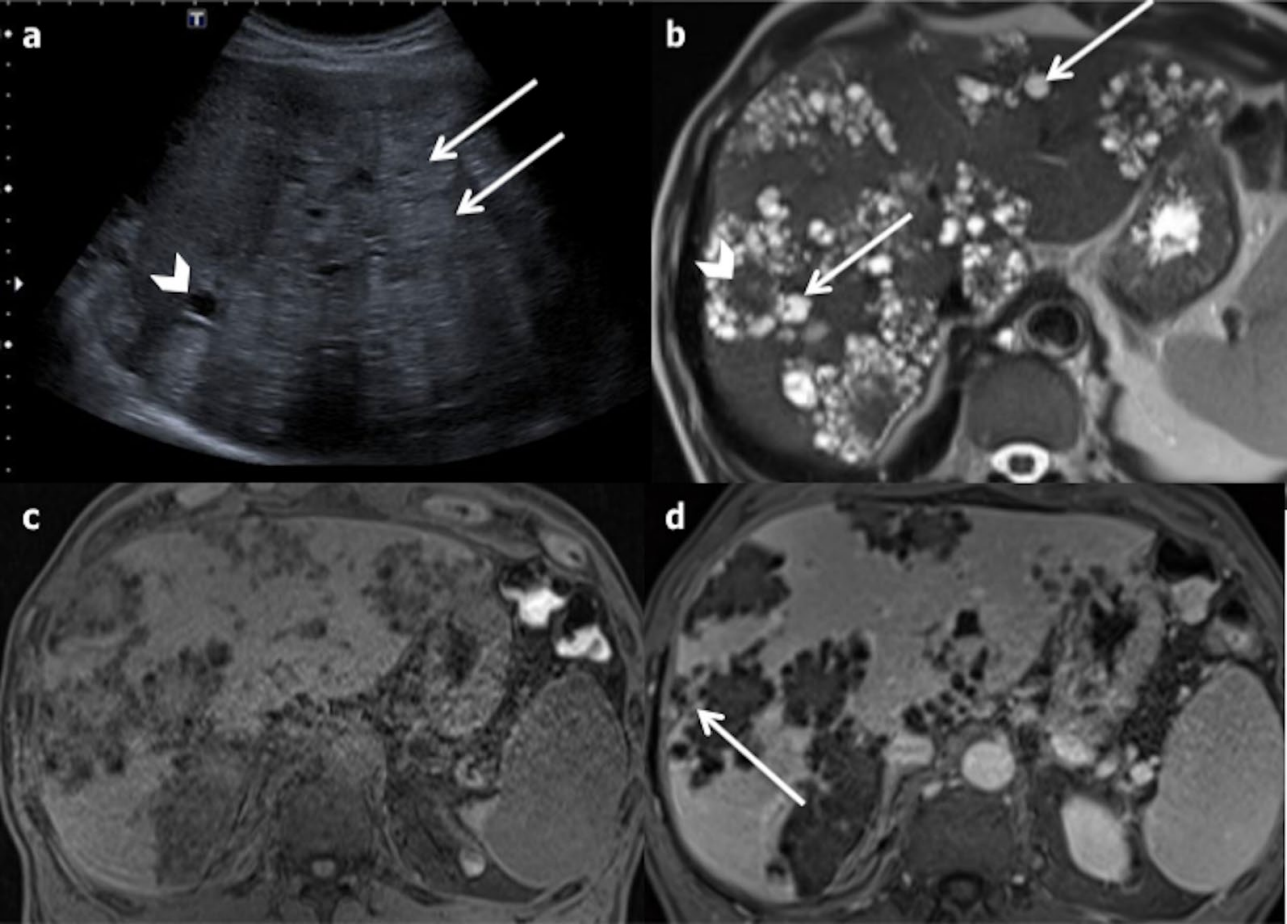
Hepatic alveolar echinococcosis in a 40-year-old male. a Ultrasonography shows multiple round hyperechoic nodules (arrows) with cystic anechoic parts (arrowhead). b On Axial T2-weighted magnetic resonance imaging, the lesions appear as multivesicular cystic masses (arrows) with a fibrous component (arrowhead). c and d On axial non-enhanced T1-fat-sat-weighted magnetic resonance imaging, the fibrous part is enhancing on late phase (arrow)
